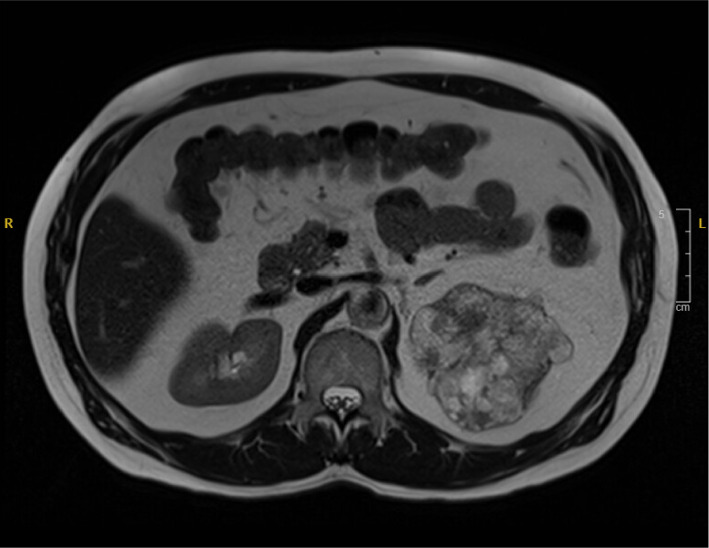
MRI shows an 8 cm, heterogeneously T_2_ hyperintense mass with internal enhancing nodular components.
